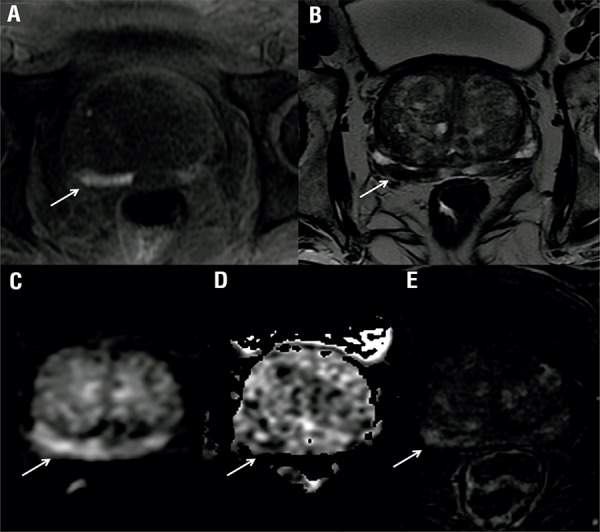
MpMRI showing suspected lesion on T1WI (a), T2WI (b), DWI (c), ADC map (d) and perfusion (e) sequences. The radiologist 1 identified lesion in the prostate right middle third and assigned as PI-RADS 5 with extra-prostatic extension. The same lesion was scored as 2 for the radiologist 2. The pathological analysis diagnosed a Gleason 6 in the right middle third. The patient was classified as having significant cancer due to PSA of 10ng/mL. In this case, the presence of blood may have limited the interpretation of the reader 2.
